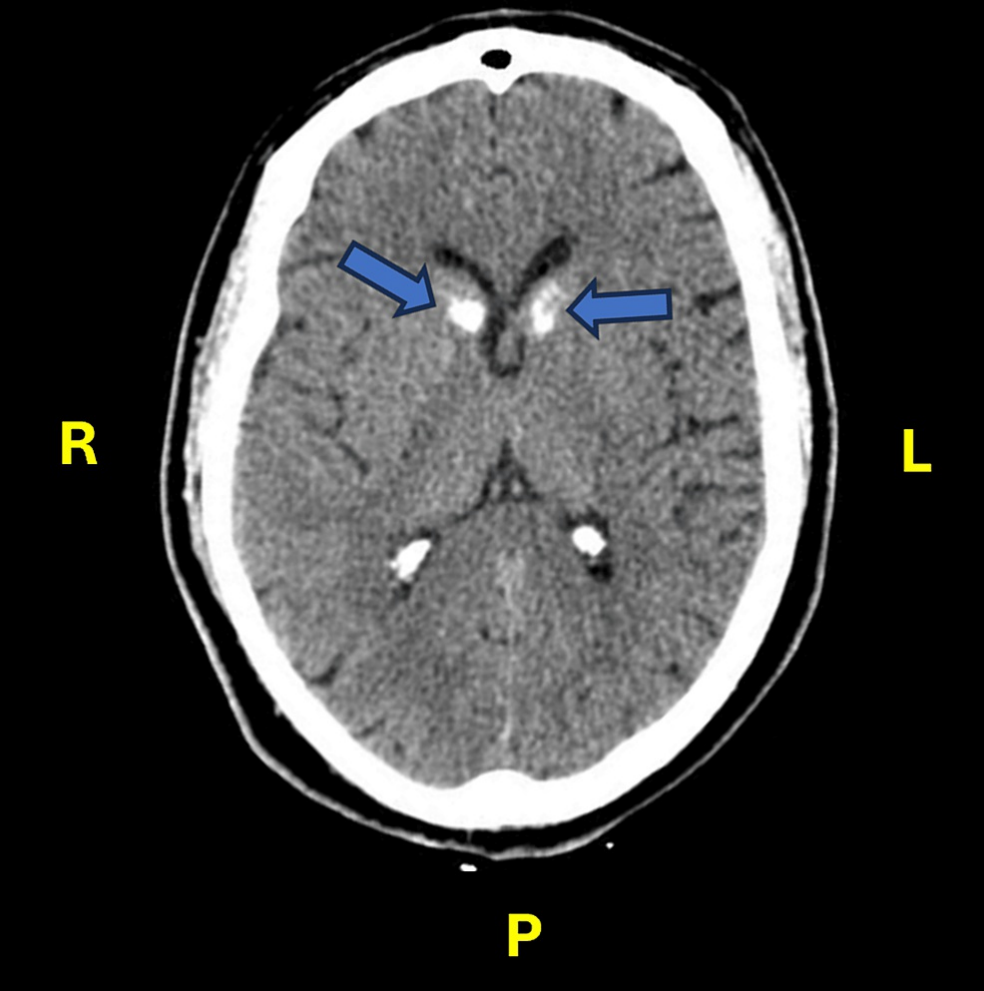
Brain CT scan image (axial section) of case 1 showing bilateral calcifications in the basal nuclei (blue arrows). CT: computed tomography
